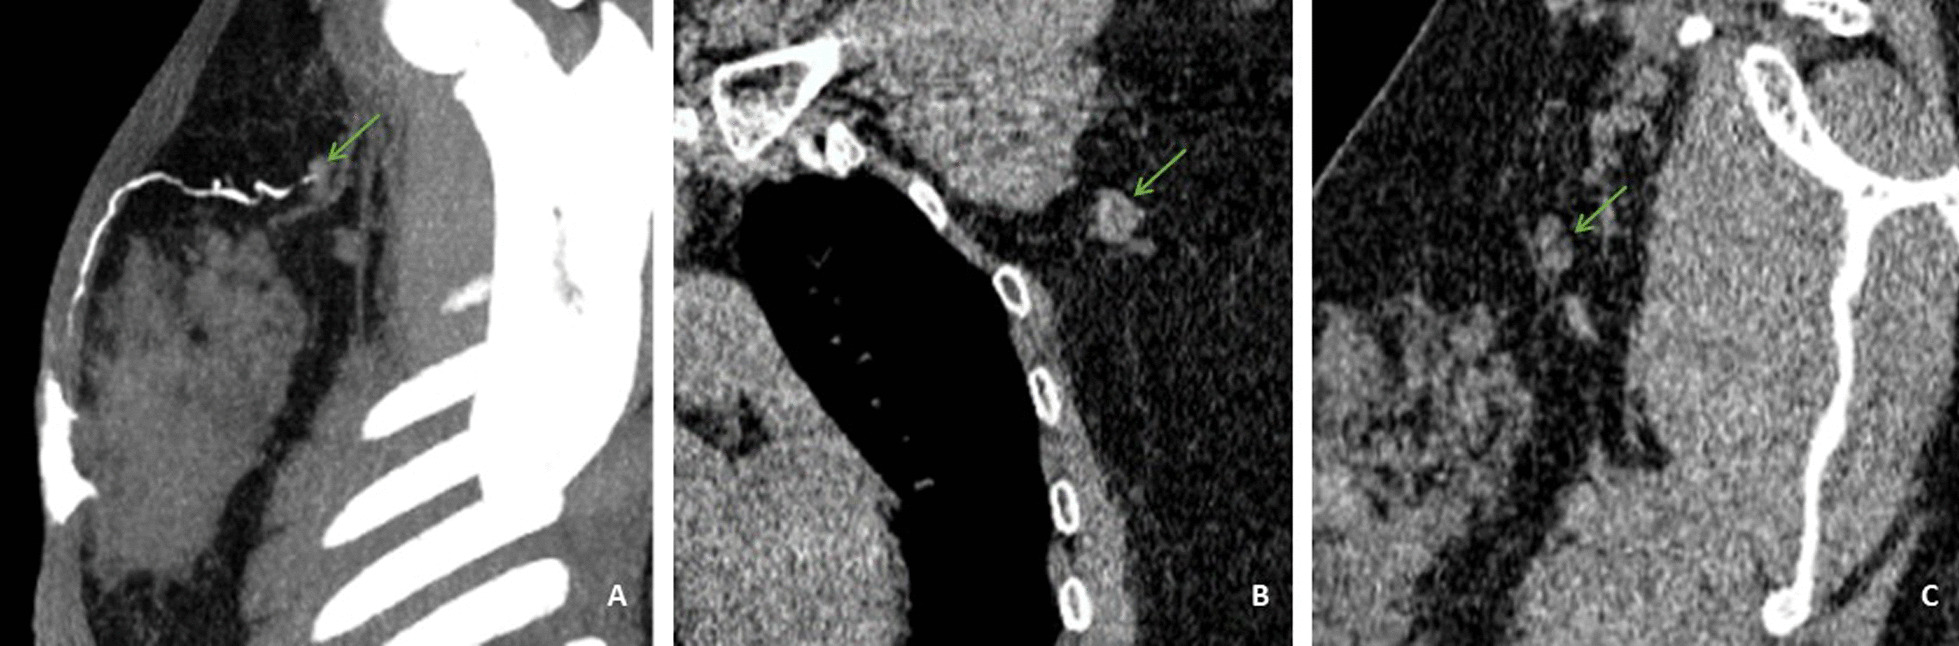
Representative multidetector-row computed tomography lymphography (MDCT-LG) images of a 59-year-old woman with left primary breast cancer (stage pT3N1, Non-specific invasive breast cancer). A The MDCT-CT 3D reconstruction image showed an sentinel lymph node was in level I. B, C Sagittal and coronal MDCT-LG image showed this lymph node with oval shape, a long-axis diameter of about 13.3 mm, and a short-axisdiameter of about 11.1 mm (green arrows) and a cortical thickness about 4.3 mm (green arrows). The patient underwent both sentinel lymph node biopsy and axillary lymph node dissection, a total of eighteen lymph nodes were removed, and sentinel lymph node biopsy removed seven lymph nodes, four of which proved to be pathologic positive
